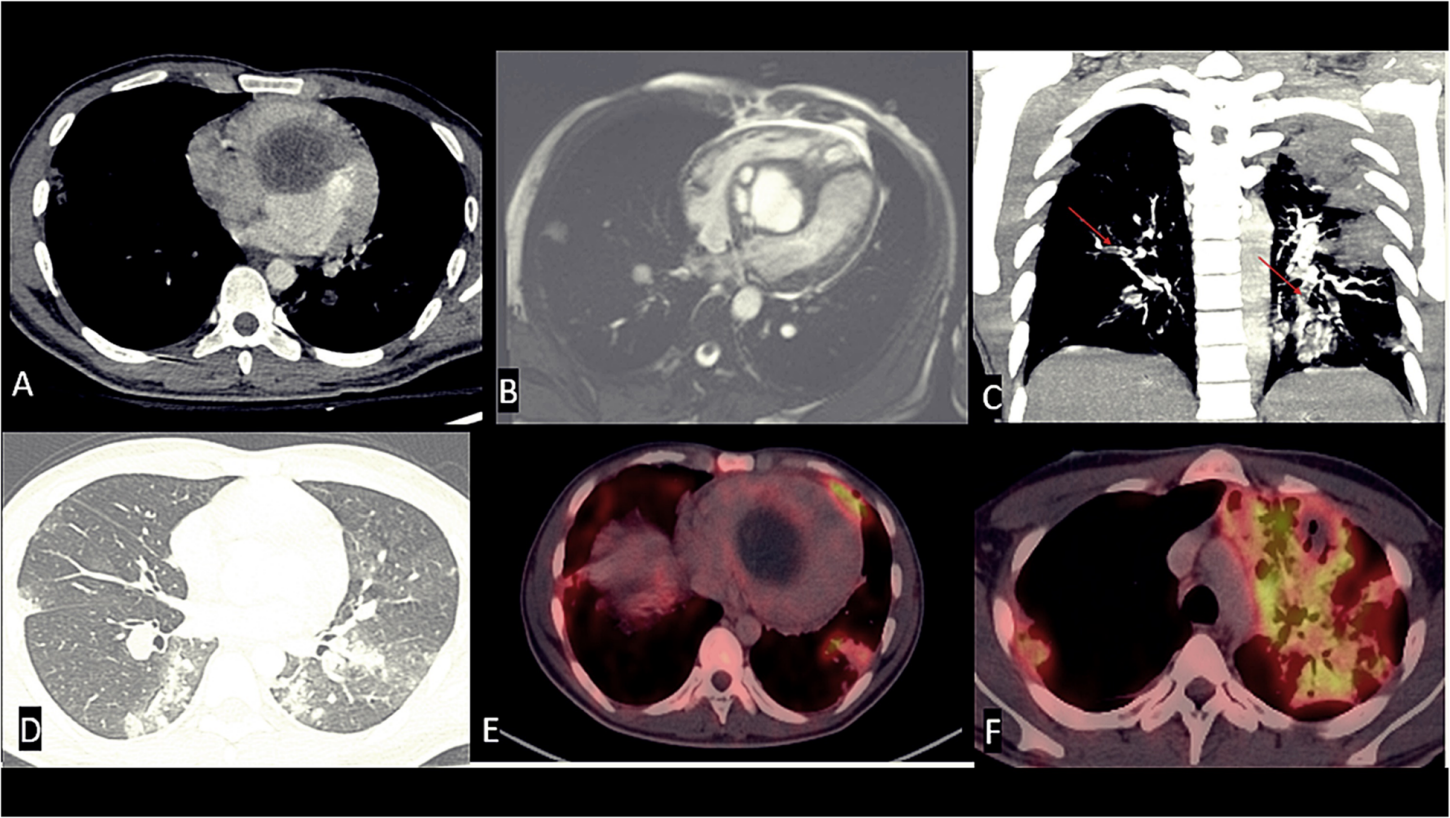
Contrast-enhanced cardiac CT (a), contrast-enhanced cardiac MR (b) axial images showing a well-defined, non-enhancing, uncalcified, multivesicular, multiloculated cyst (type II) at interventricular septum. Coronal reformatted CT images (c, d) showing segmental and subsegmental intra-pulmonary filling defects in bilateral pulmonary arteries; lung evaluation showing multiple, centri-acinar nodules with “tree in bud pattern.” PET CT showing (e) no activity at septal cystic mass (f) with avid uptake at left upper lobe consolidation
